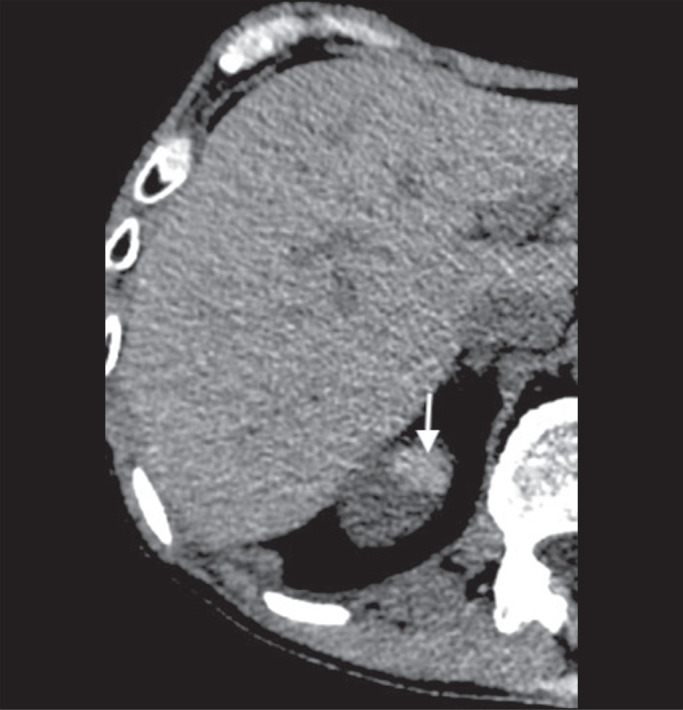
A 67-year-old male with dyspnea. Chest CT showing a hyperdense renal
